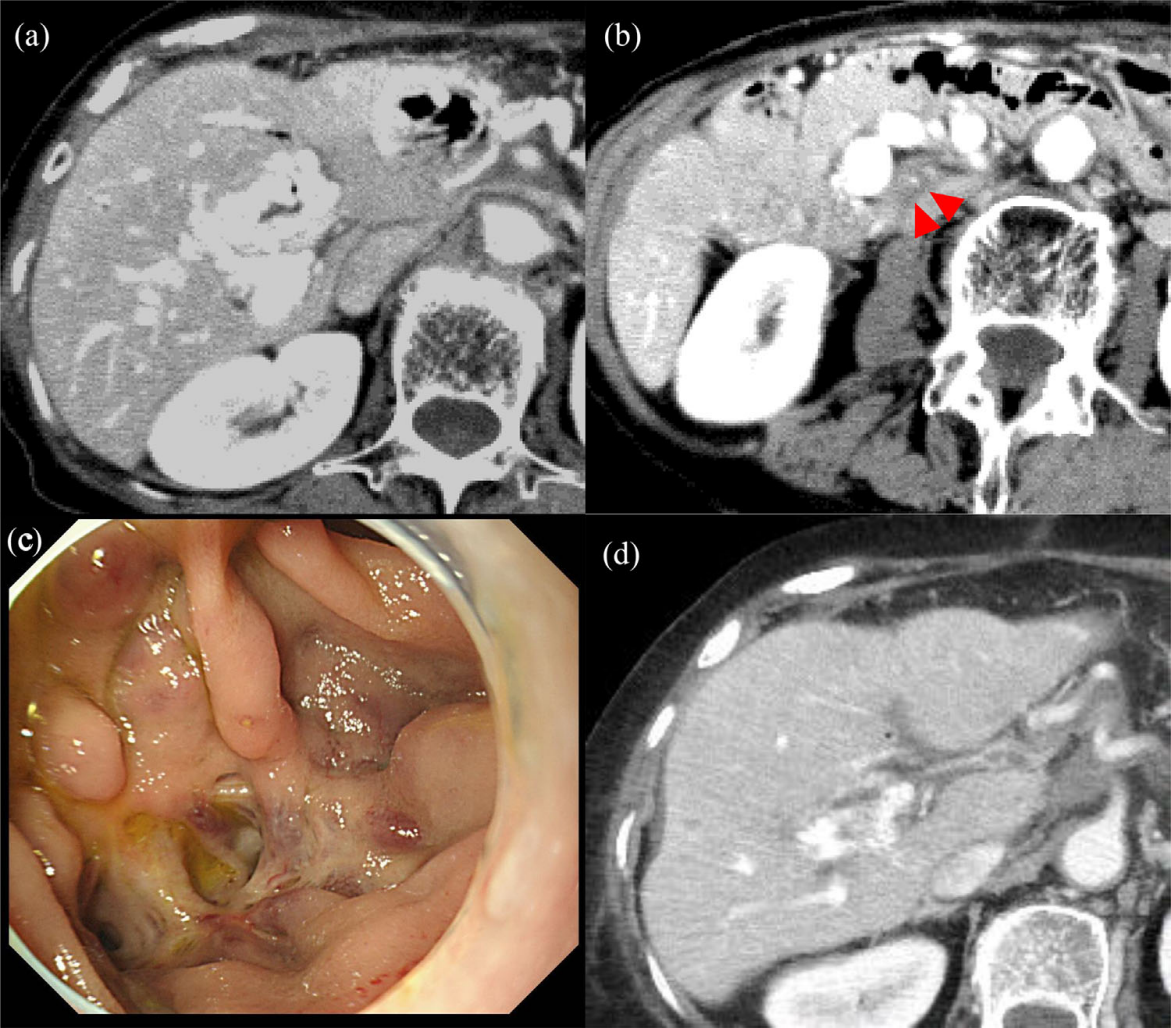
Images of case 3: (a, b) Contrast‐enhanced computed tomography (CT) showed dilated veins around the choledochojejunostomy and portal vein obstruction. (c) We found ectopic varices around the choledochojejunostomy due to portal vein obstruction. (d) CT performed 7 years after endoscopic glue injection therapy demonstrated fewer dilated veins around the choledochojejunostomy
